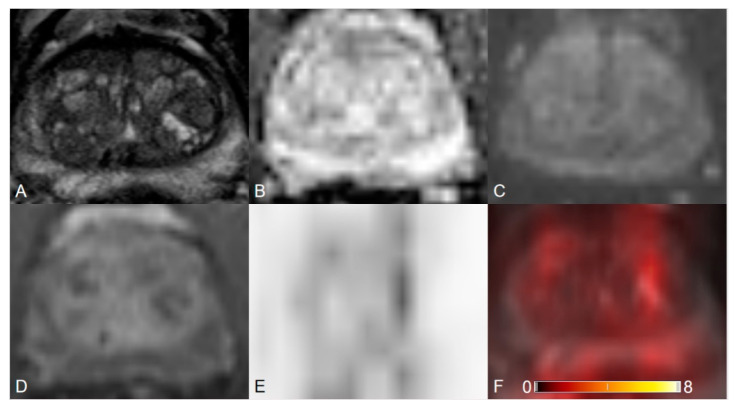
Transition zone with typical benign prostatic hyperplasia (BPH) changes. (A) Axial T2WI shows completely encapsulated “typical” nodules. (B) ADC map image presents no focal lesion with hypointense signal below the background. (C) DWI (b = 1000 s/mm^2^) shows no lesion with a markedly hyperintense signal above the background. (D) Early dynamic contrast-enhanced image presents no positive enhancement within the typical BPH nodules. T2WI = 1, DWI = 1, DCE = negative, PI-RADS assessment category = 1. (E) PET image shows inhomogeneous [^68^Ga]Ga-PSMA-11 uptake. (F) [^68^Ga]Ga-PSMA-11 PET/MRI fusion.
